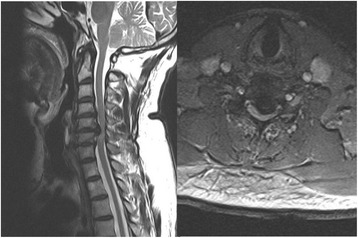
Preoperative magnetic resonance imaging showing at least three level anterior compressions of spinal cord with an occupying ratio more than 50%
